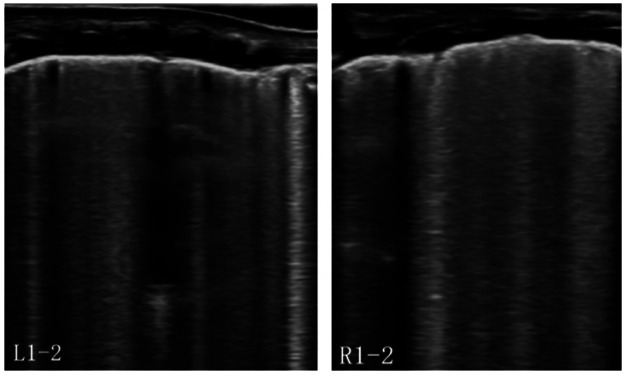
Lung ultrasound (LUS) revealed bilateral anterior pleural line irregularities, coalescent B-lines, and alveolar-interstitial syndrome (AIS). The A-lines were absent, ruling out PTX.
